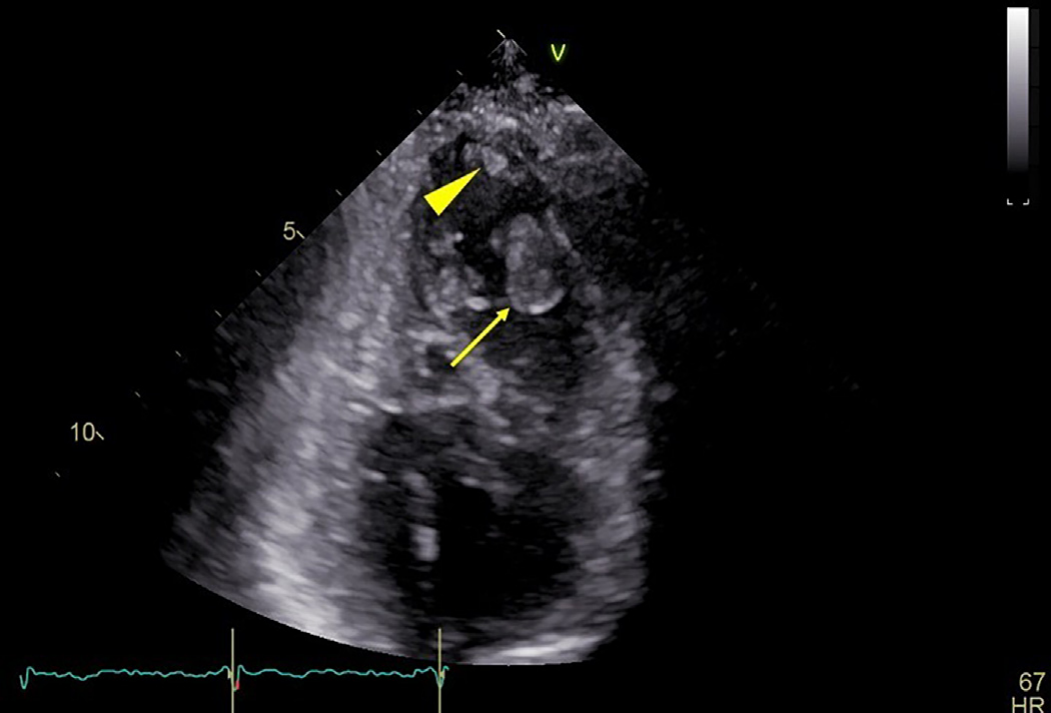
A 64-year-old man with left ventricular thrombi, detected by 2-chamber transthoracic echocardiography (*arrow* and *arrowhead*). The larger thrombus (*arrow*) was easy to identify because of its floating movement. However, the small thrombus (*arrowhead*) adherent to the myocardium was not detected at the time of examination.
